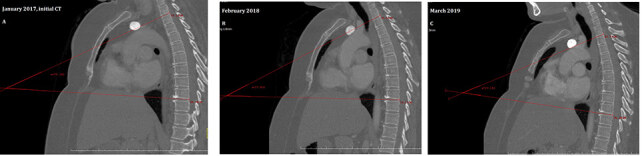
Demonstration of Cobb’s angle measurement method with computed tomography images of one of our patients in this study: 1 month after surgery (A), 1 year (B) and 2 years (C) after surgery.
